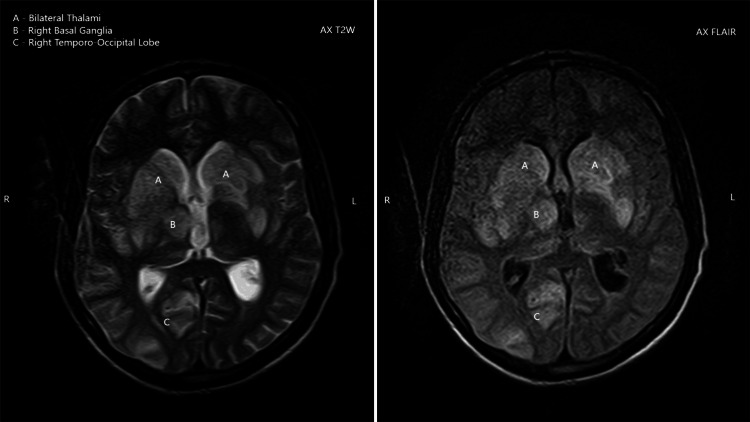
Axial T2-weighted and axial FLAIR images showing hyperintense lesions in the bilateral thalami, right basal ganglia, and right temporo-occipital white matter, with no significant mass effect, a pattern consistent with progressive multifocal leukoencephalopathy
